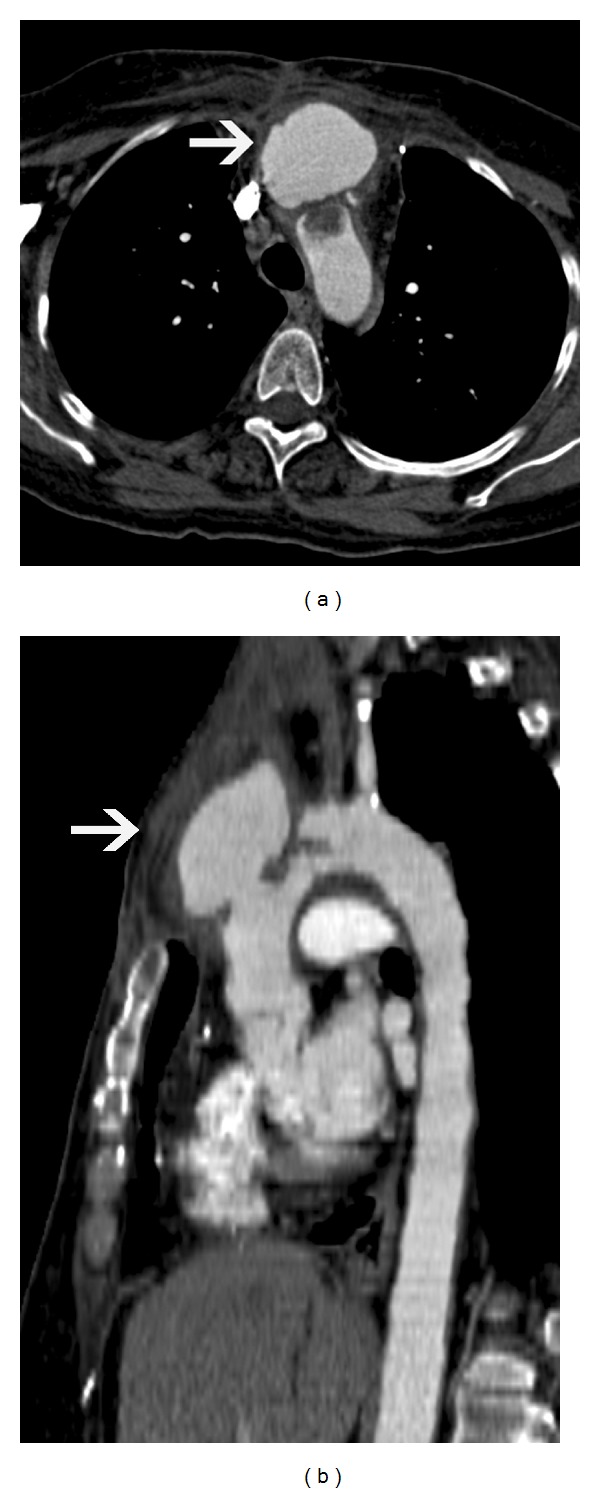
Mycotic  aneurysm—(a) axial postcontrast CT scan of a 72-year-old male shows a large saccular pseudoaneurysm (arrow) arising from the anterior aspect of the distal ascending aorta, which is anteriorly eroding the sternum. (b) Sagittal postcontrast CT scan in the same patient shows the focal mycotic pseudoaneurysm (arrow) that is arising from the anterior aspect of ascending aorta and eroding the anterior sternum.
